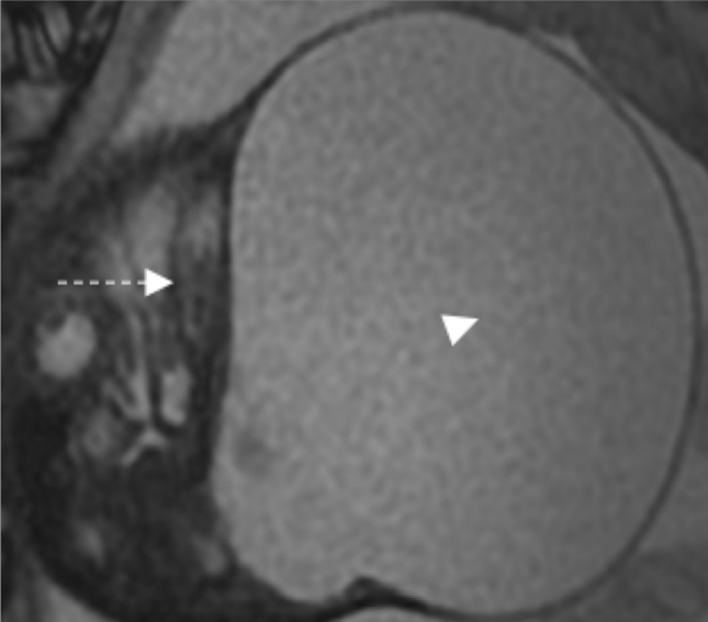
Fetal MRI at 31 weeks gestation. Axial T2-weighted fetal MRI demonstrates interval increase in the size of the large cystic mass (arrowhead) with increased mass effect on the left orbit (dotted arrow)
